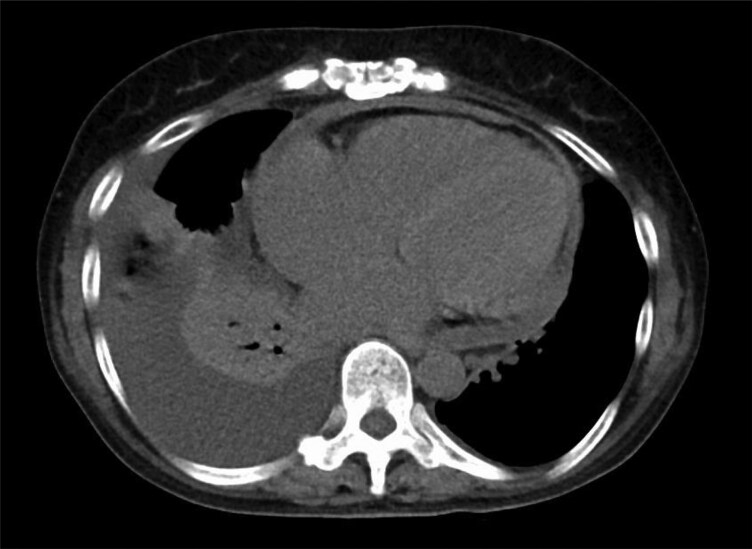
A plain computed tomography scan showing thickening of the pericardium and a small amount of pericardial effusion.
